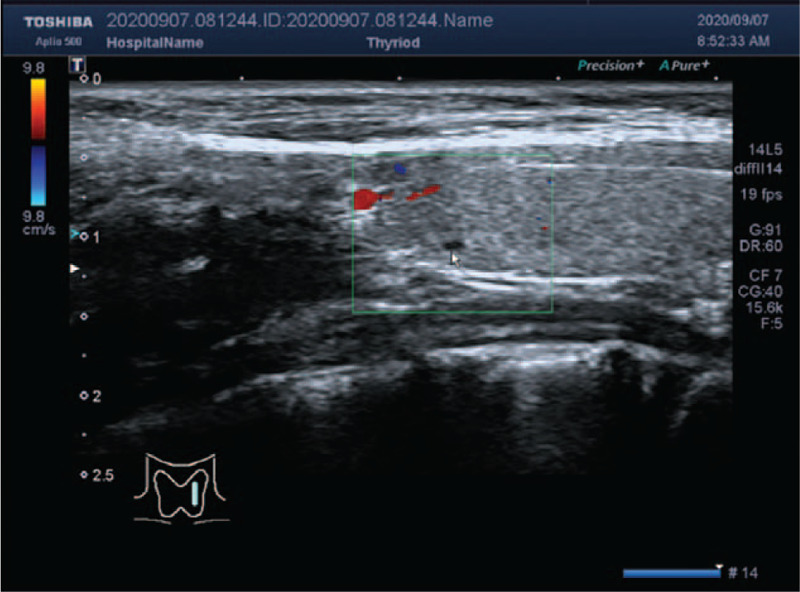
Thyroid gland ultrasound examination in the patient. The white arrow indicates a weak-echo nodule in the left lobe of her thyroid gland.
